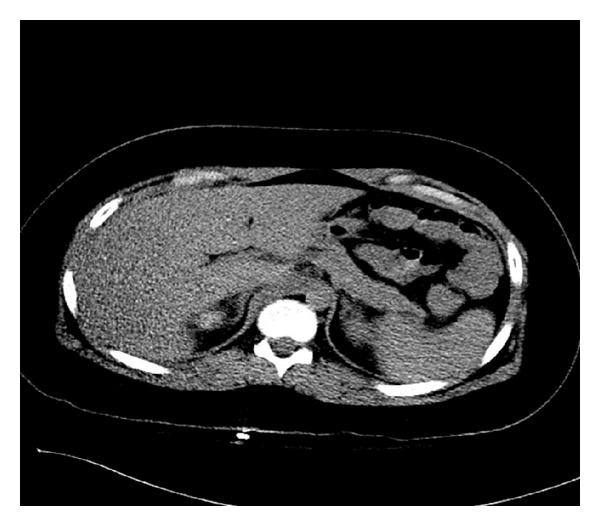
Abdominal CT scan showing no hepatic parenchymal injury.
